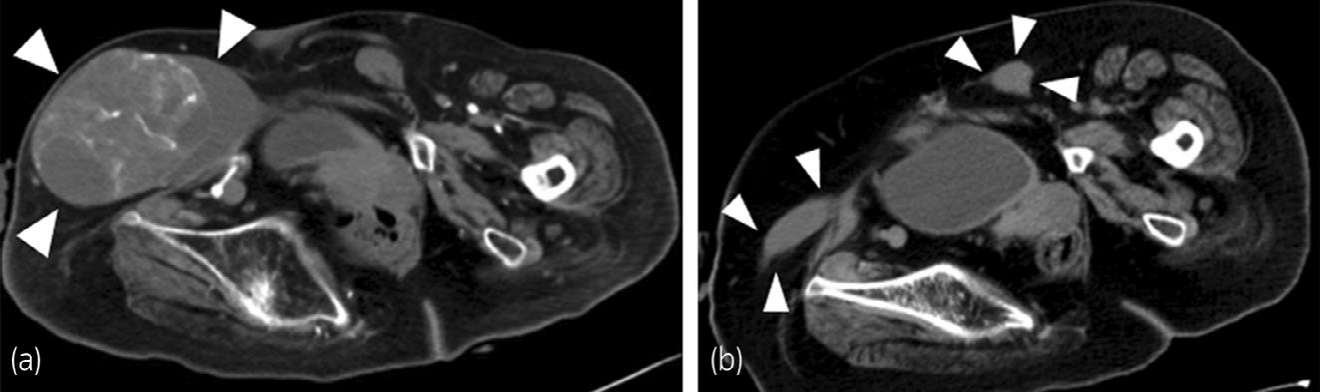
Pelvic CT images. (a) A 10‐cm well‐circumscribed oval tumor (white arrowheads). (b) An image of the bilateral UDT (white arrowheads) obtained 4 years ago.
